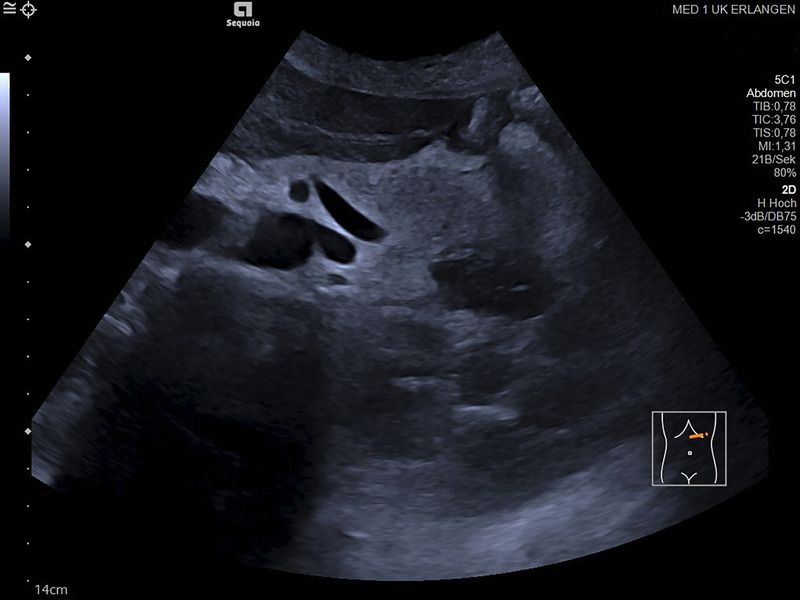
Abdominal ultrasound 3 days post-intervention.
